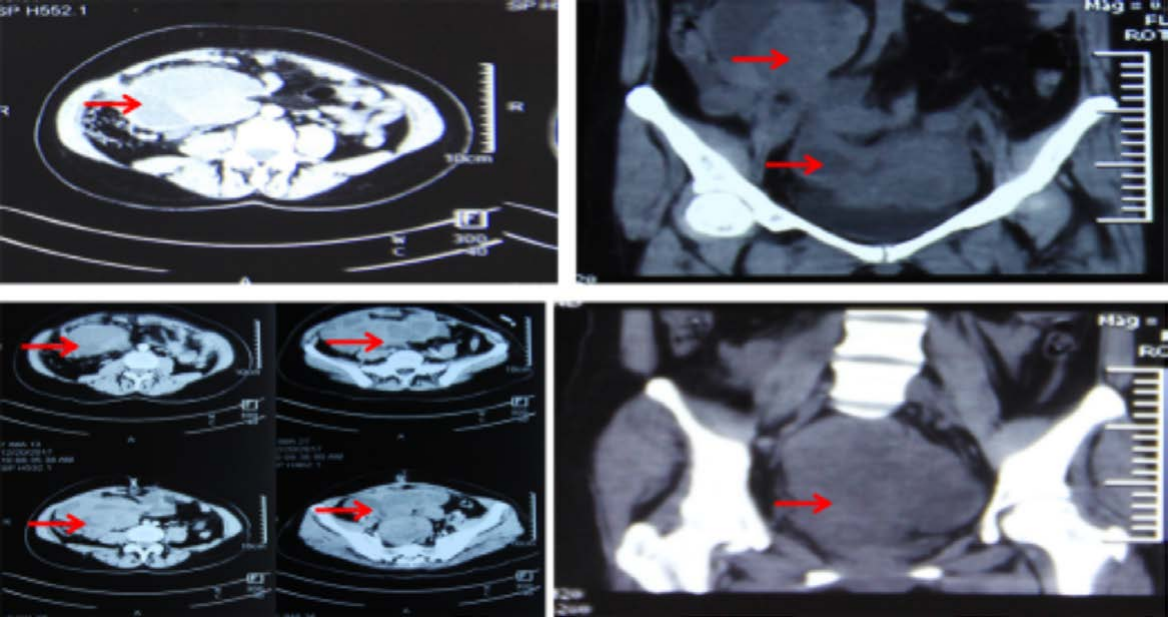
Pelvic contrast-enhanced computed tomography shows multiple cystic spaces occupying lesions in the pelvis, changes after hysterectomy, and abnormal enhancement of soft tissue nodules in the incision (December 2017).
